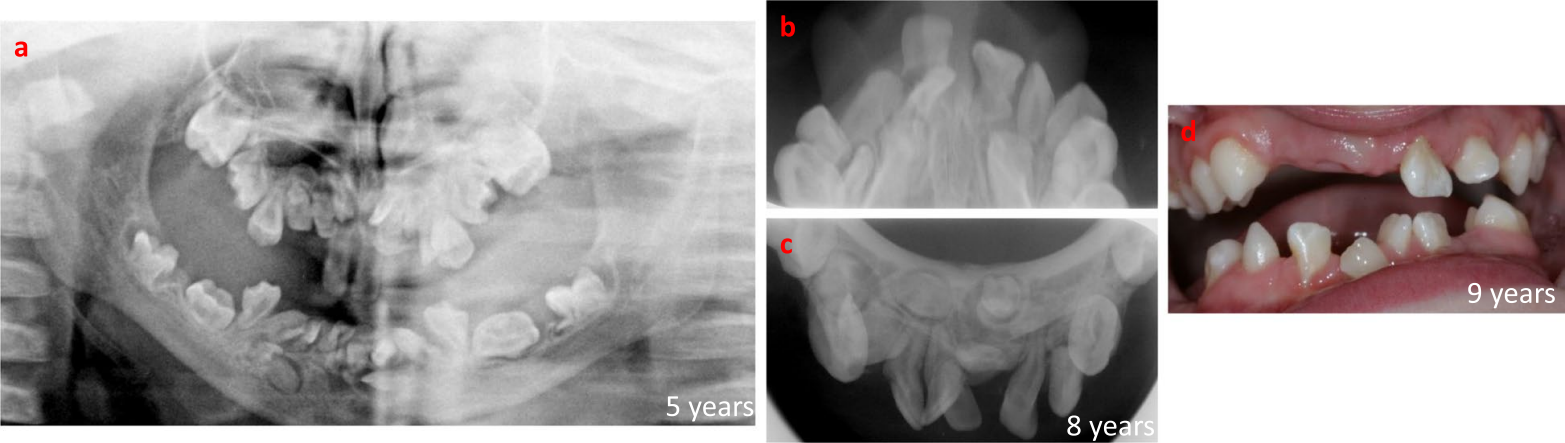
Overviews of the dentition before any dental treatment at ages 5, 8 and 9 years. a Panoramic radiograph at 5 years. Radiographic overview at 8 years of b upper jaw and c lower jaw. d Photographic overview of the dentition at 9 years
